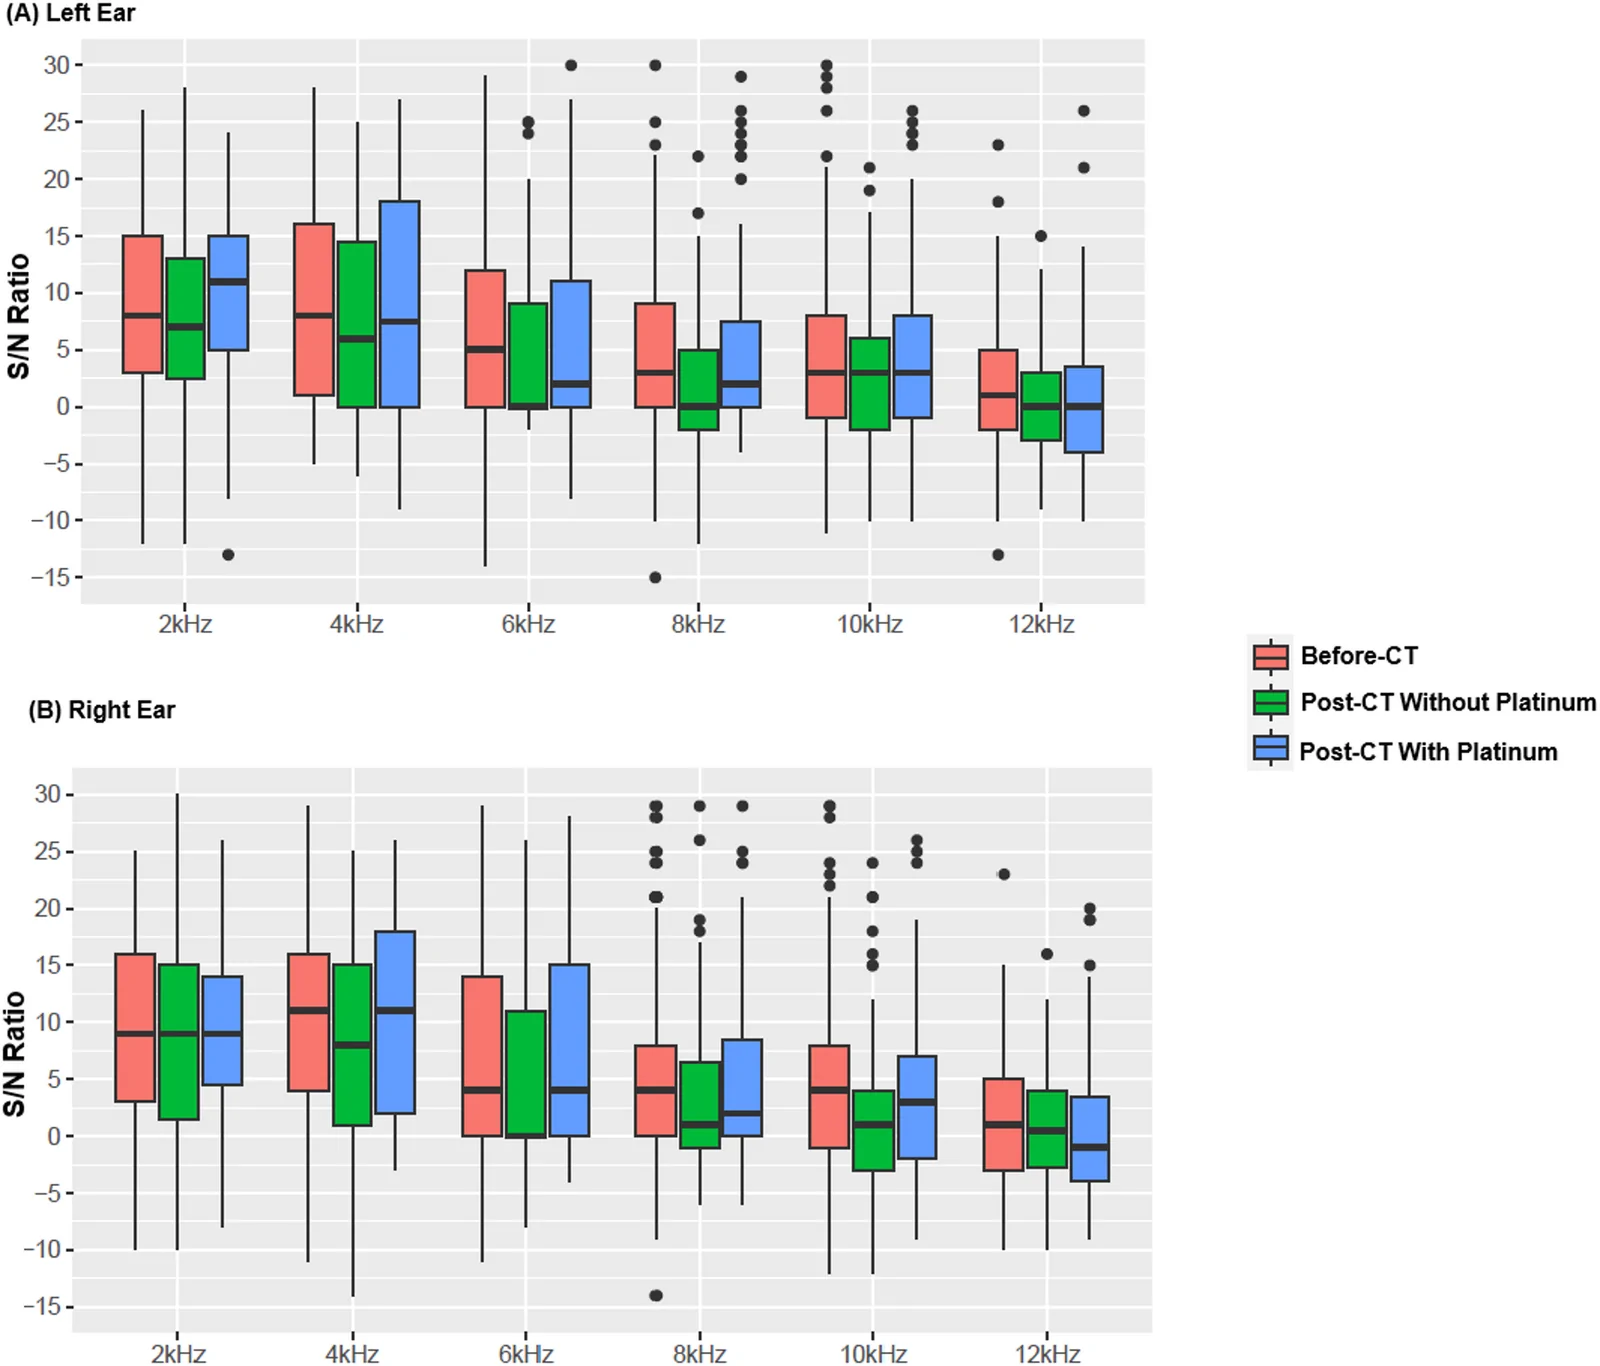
Comparison of DPOAE pre-CT and post-CT chemotherapy in the left ear (A) and right ear (B)
